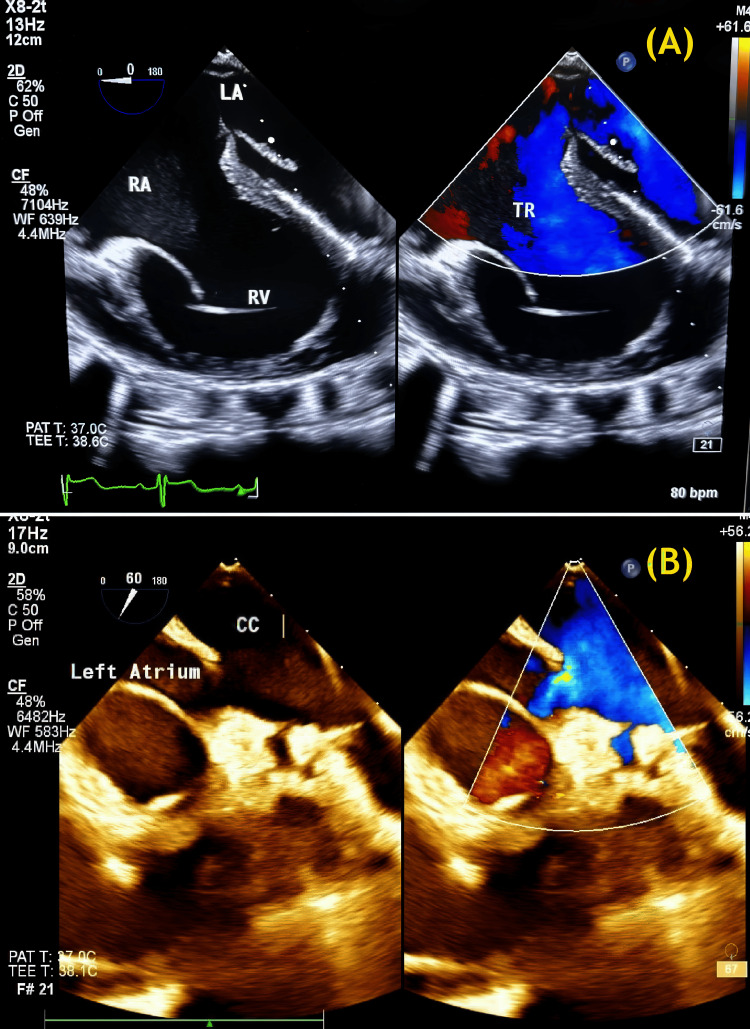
Postoperative TEE demonstrated (A) wide communication between the CC of the pulmonary veins and the LA; and (B) the connection created between the common chamber and the LA. CC: Common chamber; LA: Left atrium; TEE: Transesophageal echocardiography. Image credit: Dr. Sambhunath Das
